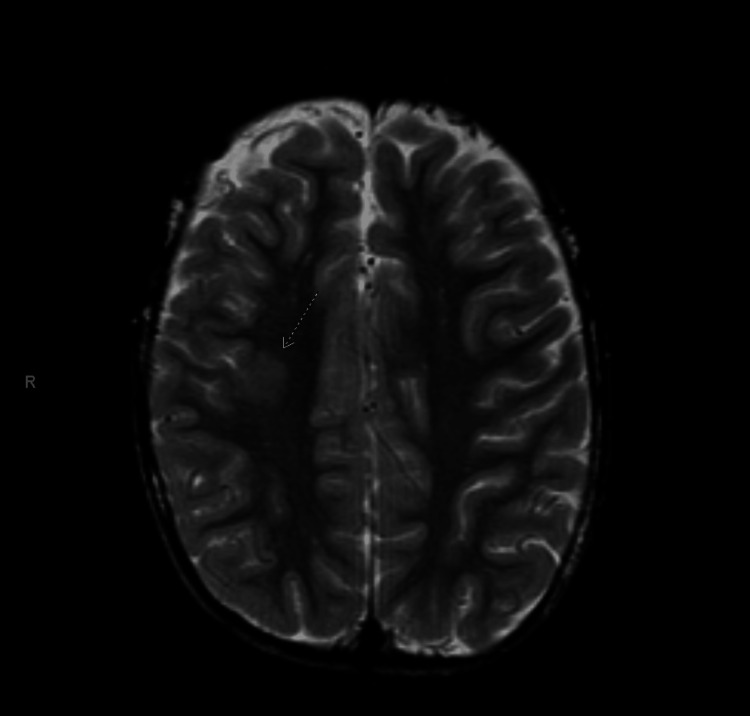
Axial T2-weighted MRI of the brain demonstrating asymmetric cortical thickening of the posterior right frontal lobe, indicated by the arrow, which is consistent with the patient’s previously diagnosed right frontal lobe cortical dysplasia.
